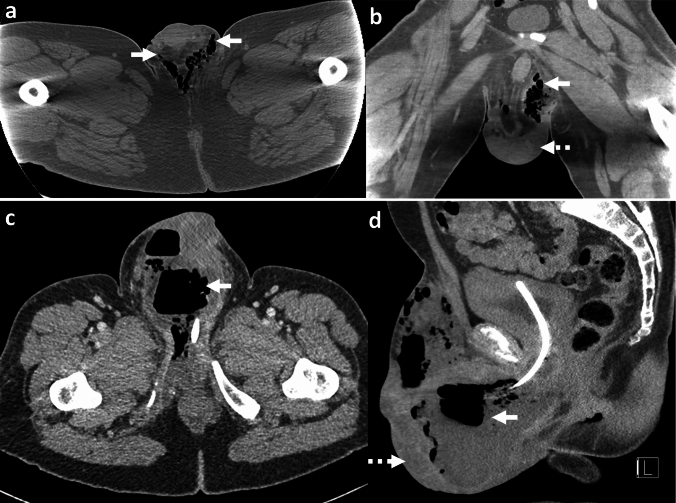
Fournier’s gangrene. a, b A 35-year-old diabetic with scrotal pain and erythema. CT images show air in the scrotum (solid white arrows, a, b) with scrotal skin thickening (dotted white arrow, b). c, d A 55-year-old diabetic with urosepsis, scrotal swelling, pain, and erythema. CT images show air in the scrotum with multiple air-fluid levels (solid white arrow, c) with extensive scrotal wall edema (dotted white arrow, d)
